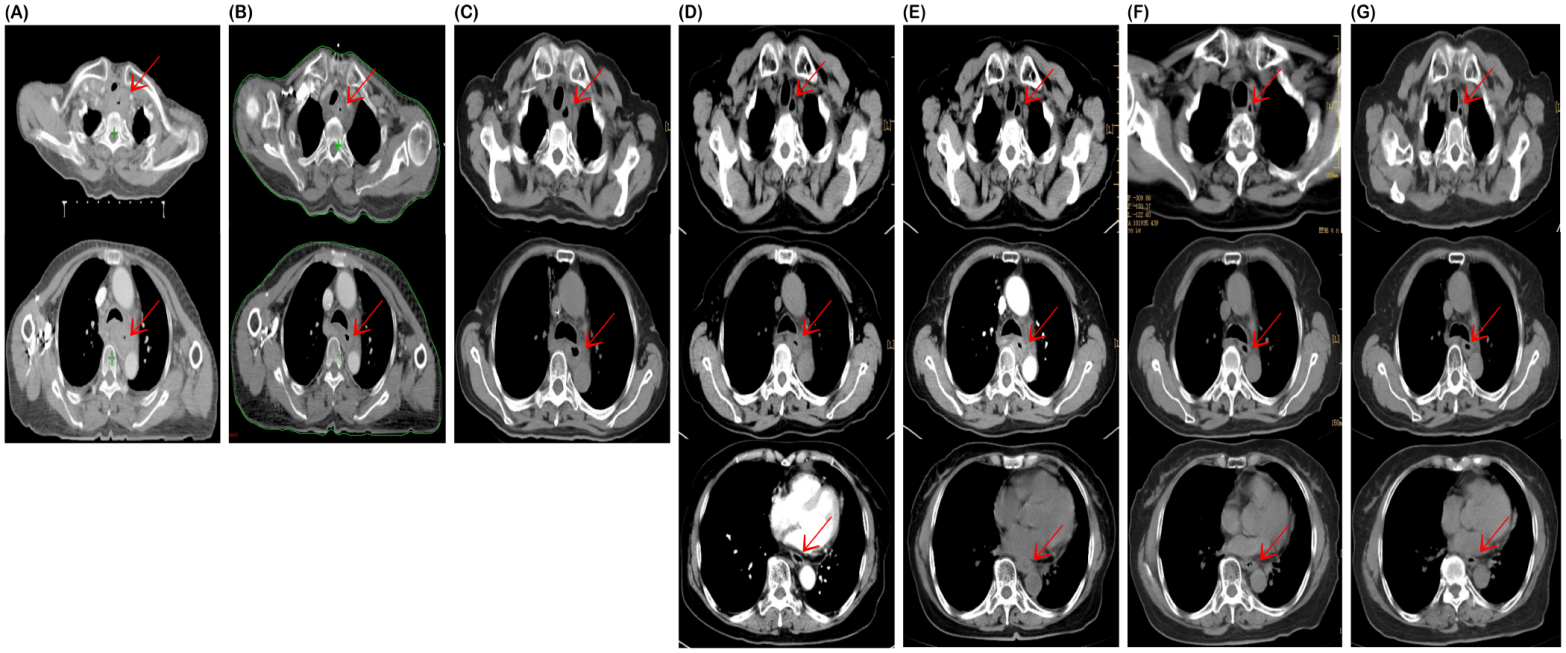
Computed tomography (CT) at the start of treatment on October 20, 2021 (A), offline ART-based CT on November 17, 2021 (B), and post-radiotherapy assessment CT on January 29, 2022 (C), showed progressive tumor shrinkage. Computed tomography (CT) on June 16, 2022 (D), after maintenance therapy showed stable disease (SD); on March 24, 2023 (E), thoracic lesions remained stable, but esophageal thickening indicated tumor progression. Following treatment with sintilimab and anlotinib, chest CT scans on January 23, 2024 (F), and April 16, 2024 (G), indicated continued partial response.
